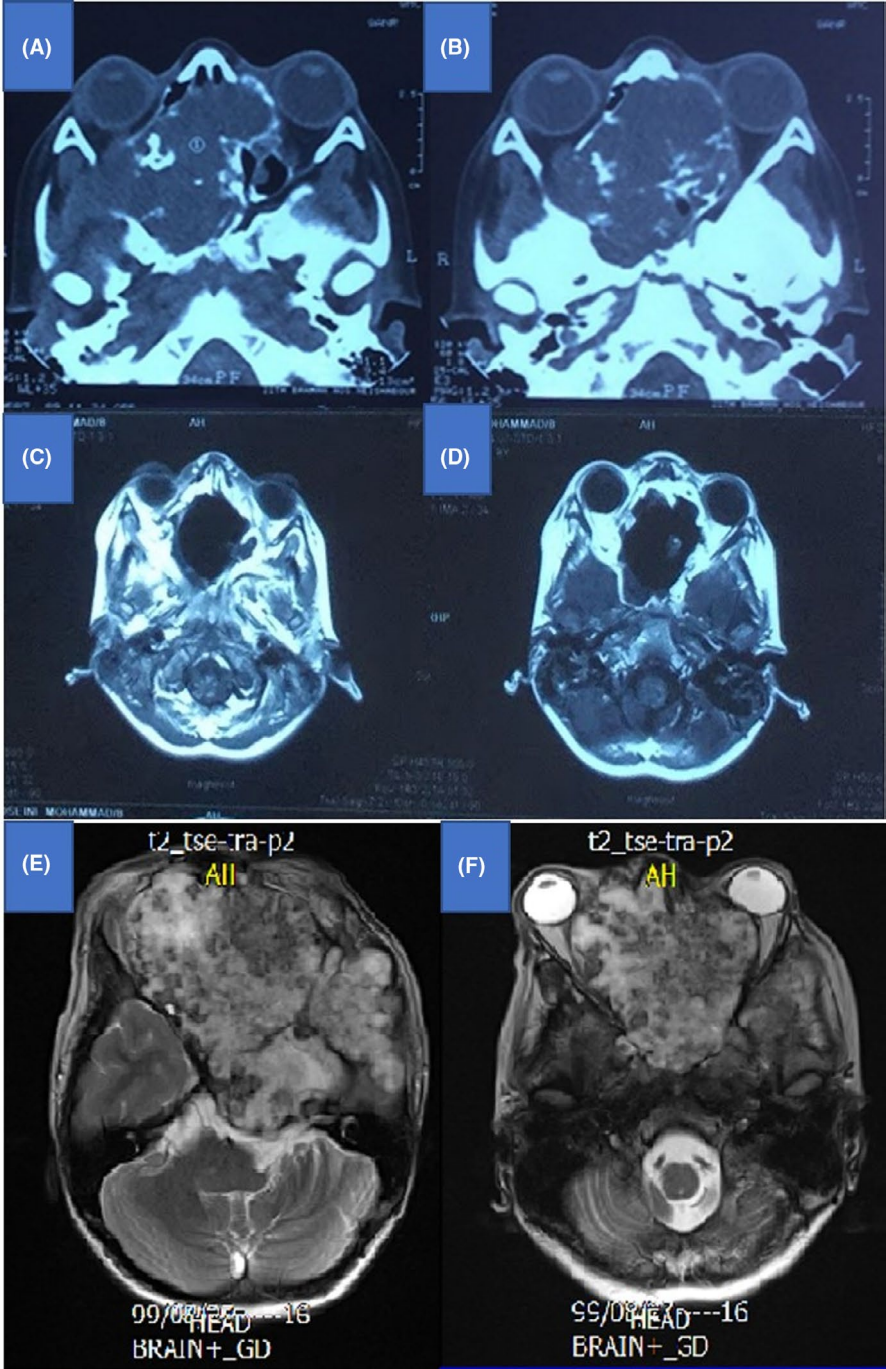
(A) Initial CT imaging indicating the diagnosis of skull base tumor. (B) Post‐surgical changes and complete excision demonstrated in the first post‐surgical CT imaging. (C) Final MRI imaging indicating a large heterogeneous tumoral mass which remained stable after radiation therapy
